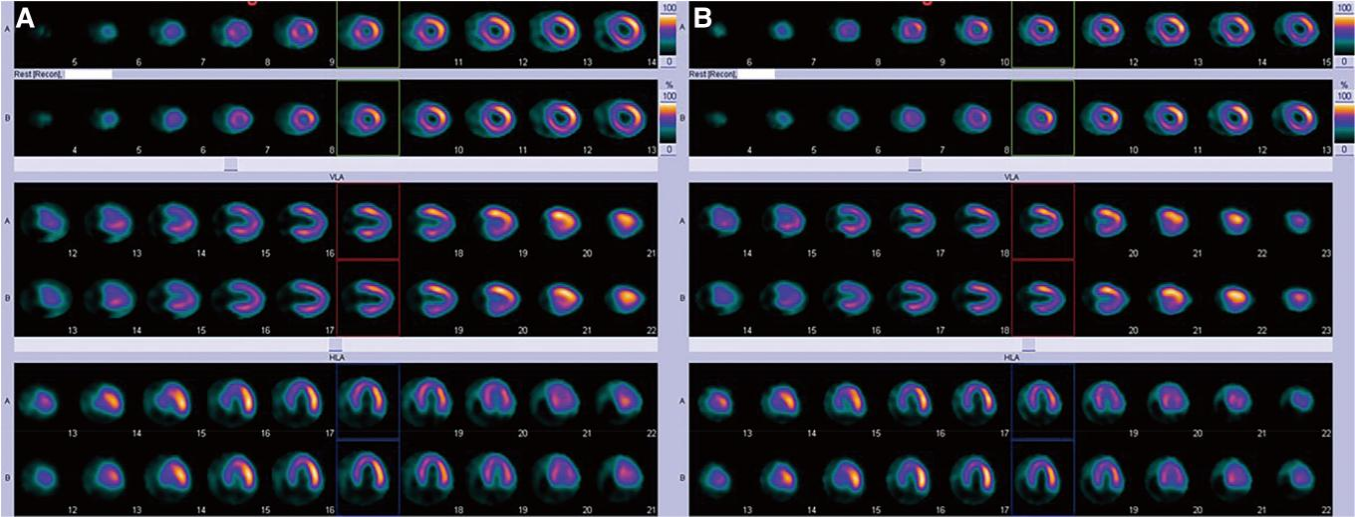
Stress myocardial perfusion scintigraphy before and after transcatheter embolization. Exercise stress myocardial perfusion imaging shows no evidence of inducible ischaemia before (*A*) and after (*B*) fistula embolization. No regional perfusion defects or exercise-induced ischaemic changes were detected in either study.
